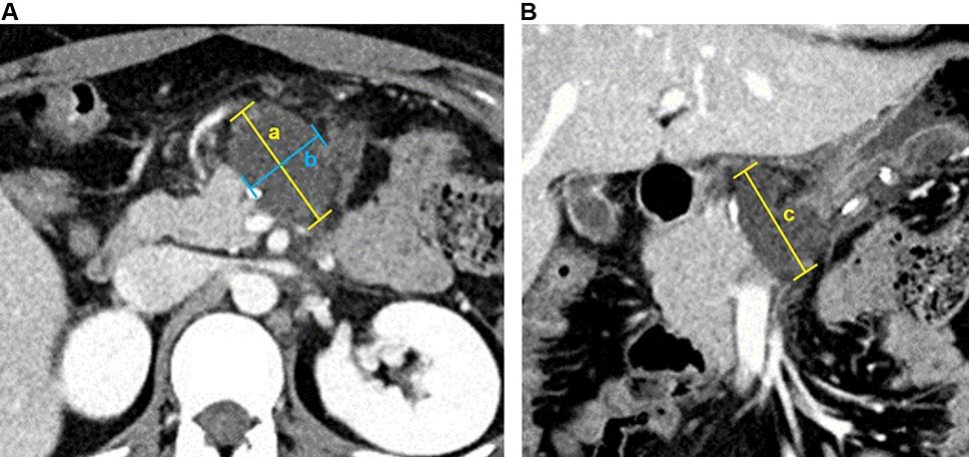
Measurement of the postoperative fluid collection on a computed tomography (CT) image. Among the multiple cut images of the postoperative CT scan, the image with the largest fluid collection was selected. The length (*a*) and width (*b*) on the axial view (A) and the height (*c*) on the coronal view (B) were measured. The volume of the postoperative fluid collection was calculated by multiplying the length, width, and height (volume of fluid collection = *a* × *b* × *c*, mm^3^)
